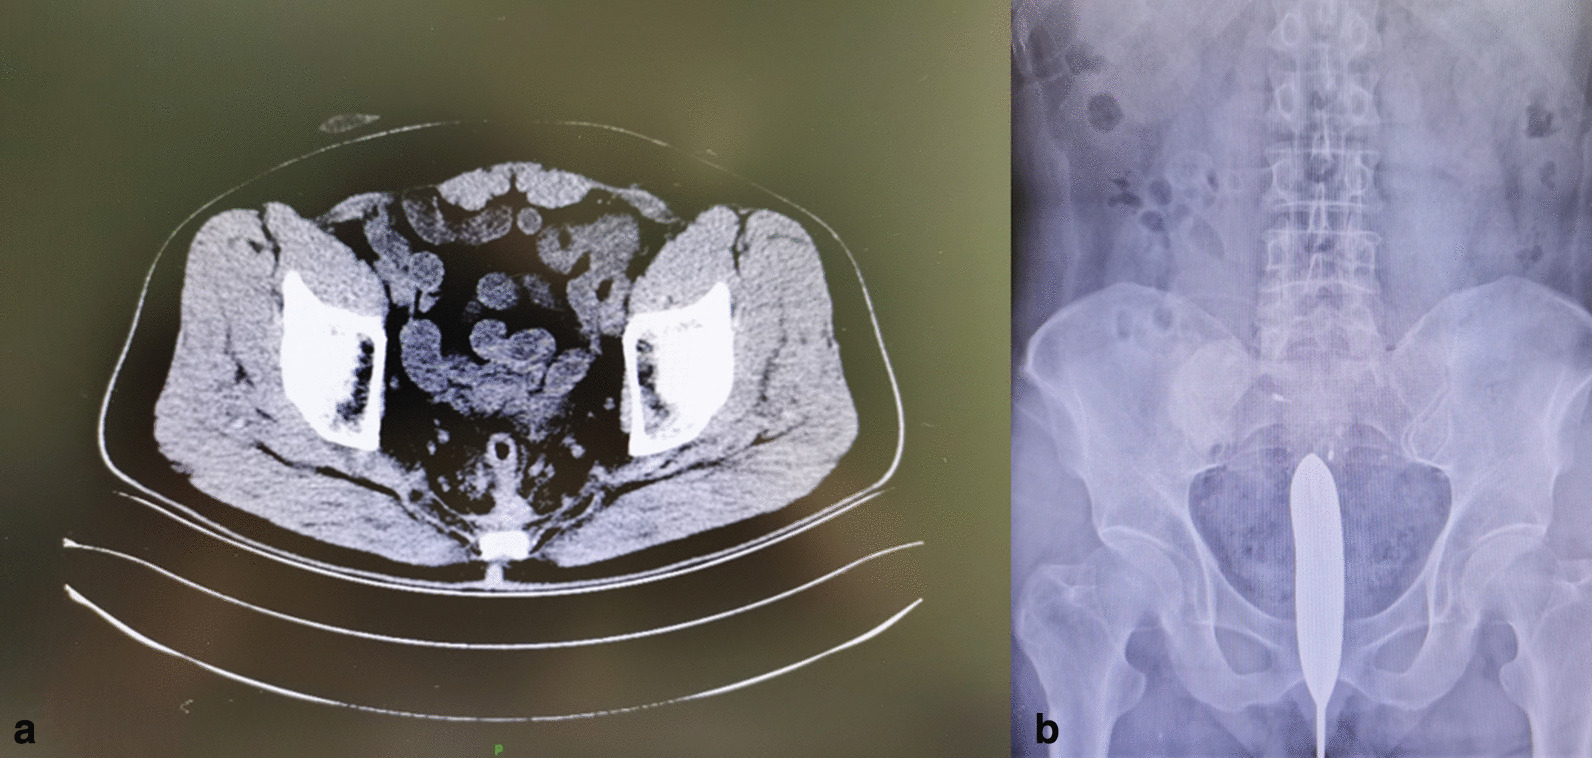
The imaging scanning of the patient. a Abdominal enhanced CT showed that the intestinal lumen above the anastomotic stoma was narrow and adhered to the anterior sacral fascia. b In the barium enema examination, the anastomotic stoma in rectum was completely closed and separated
